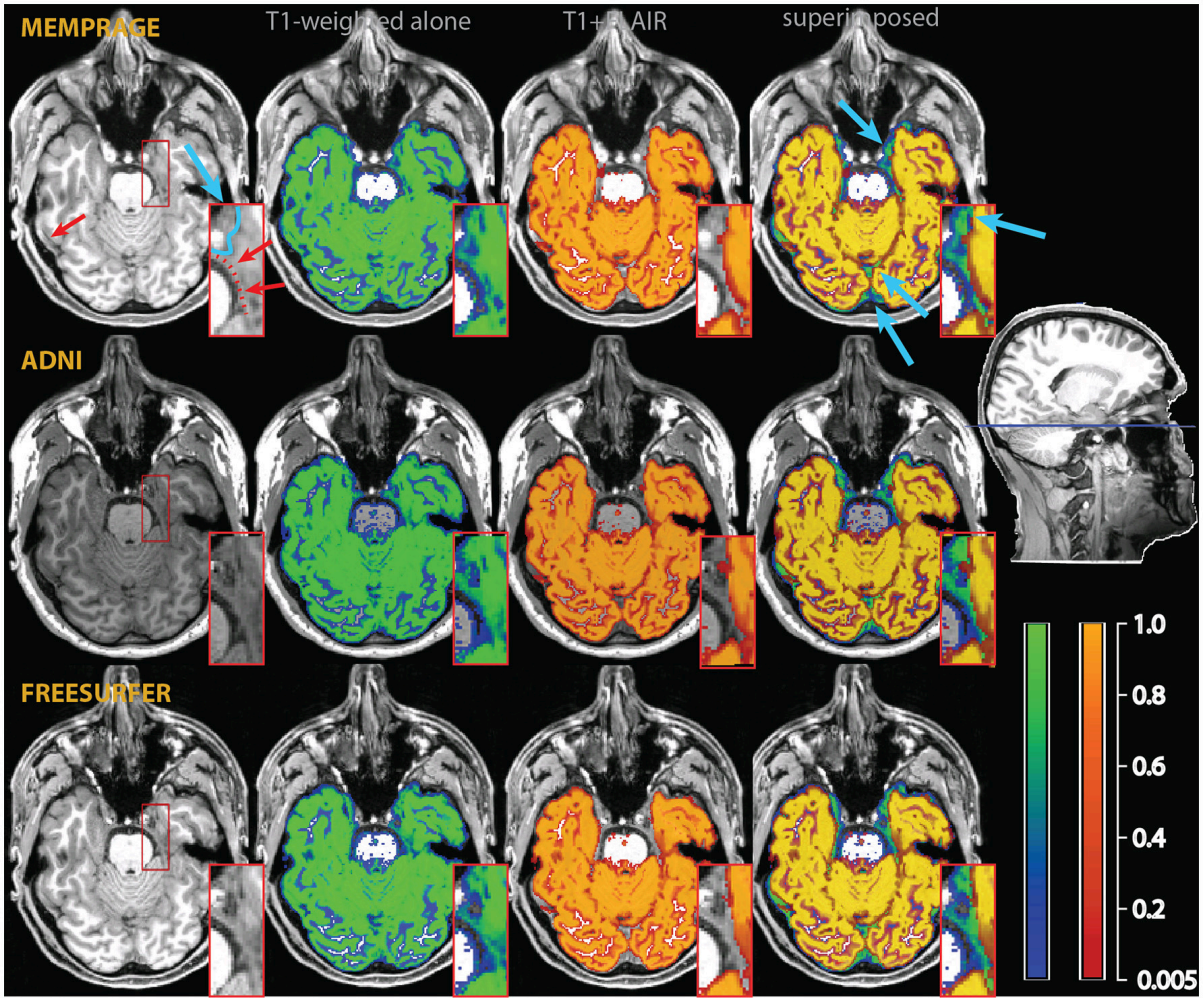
Example of a misclassification of vessels as gray matter in the medial aspect of the temporal lobe (red arrows and dotted line in the inset in the top left). As in Figure 2, the segmentation of the T1-weighted images alone is shown as a blue-green overlay, and the multimodal segmentation with the added FLAIR images in red-orange. Light blue arrows point to differences in the classification of extracerebral tissue. The insets show an enlarged section of the medial right temporal lobe, where the improvements due to the multimodal segmentation are apparent.
